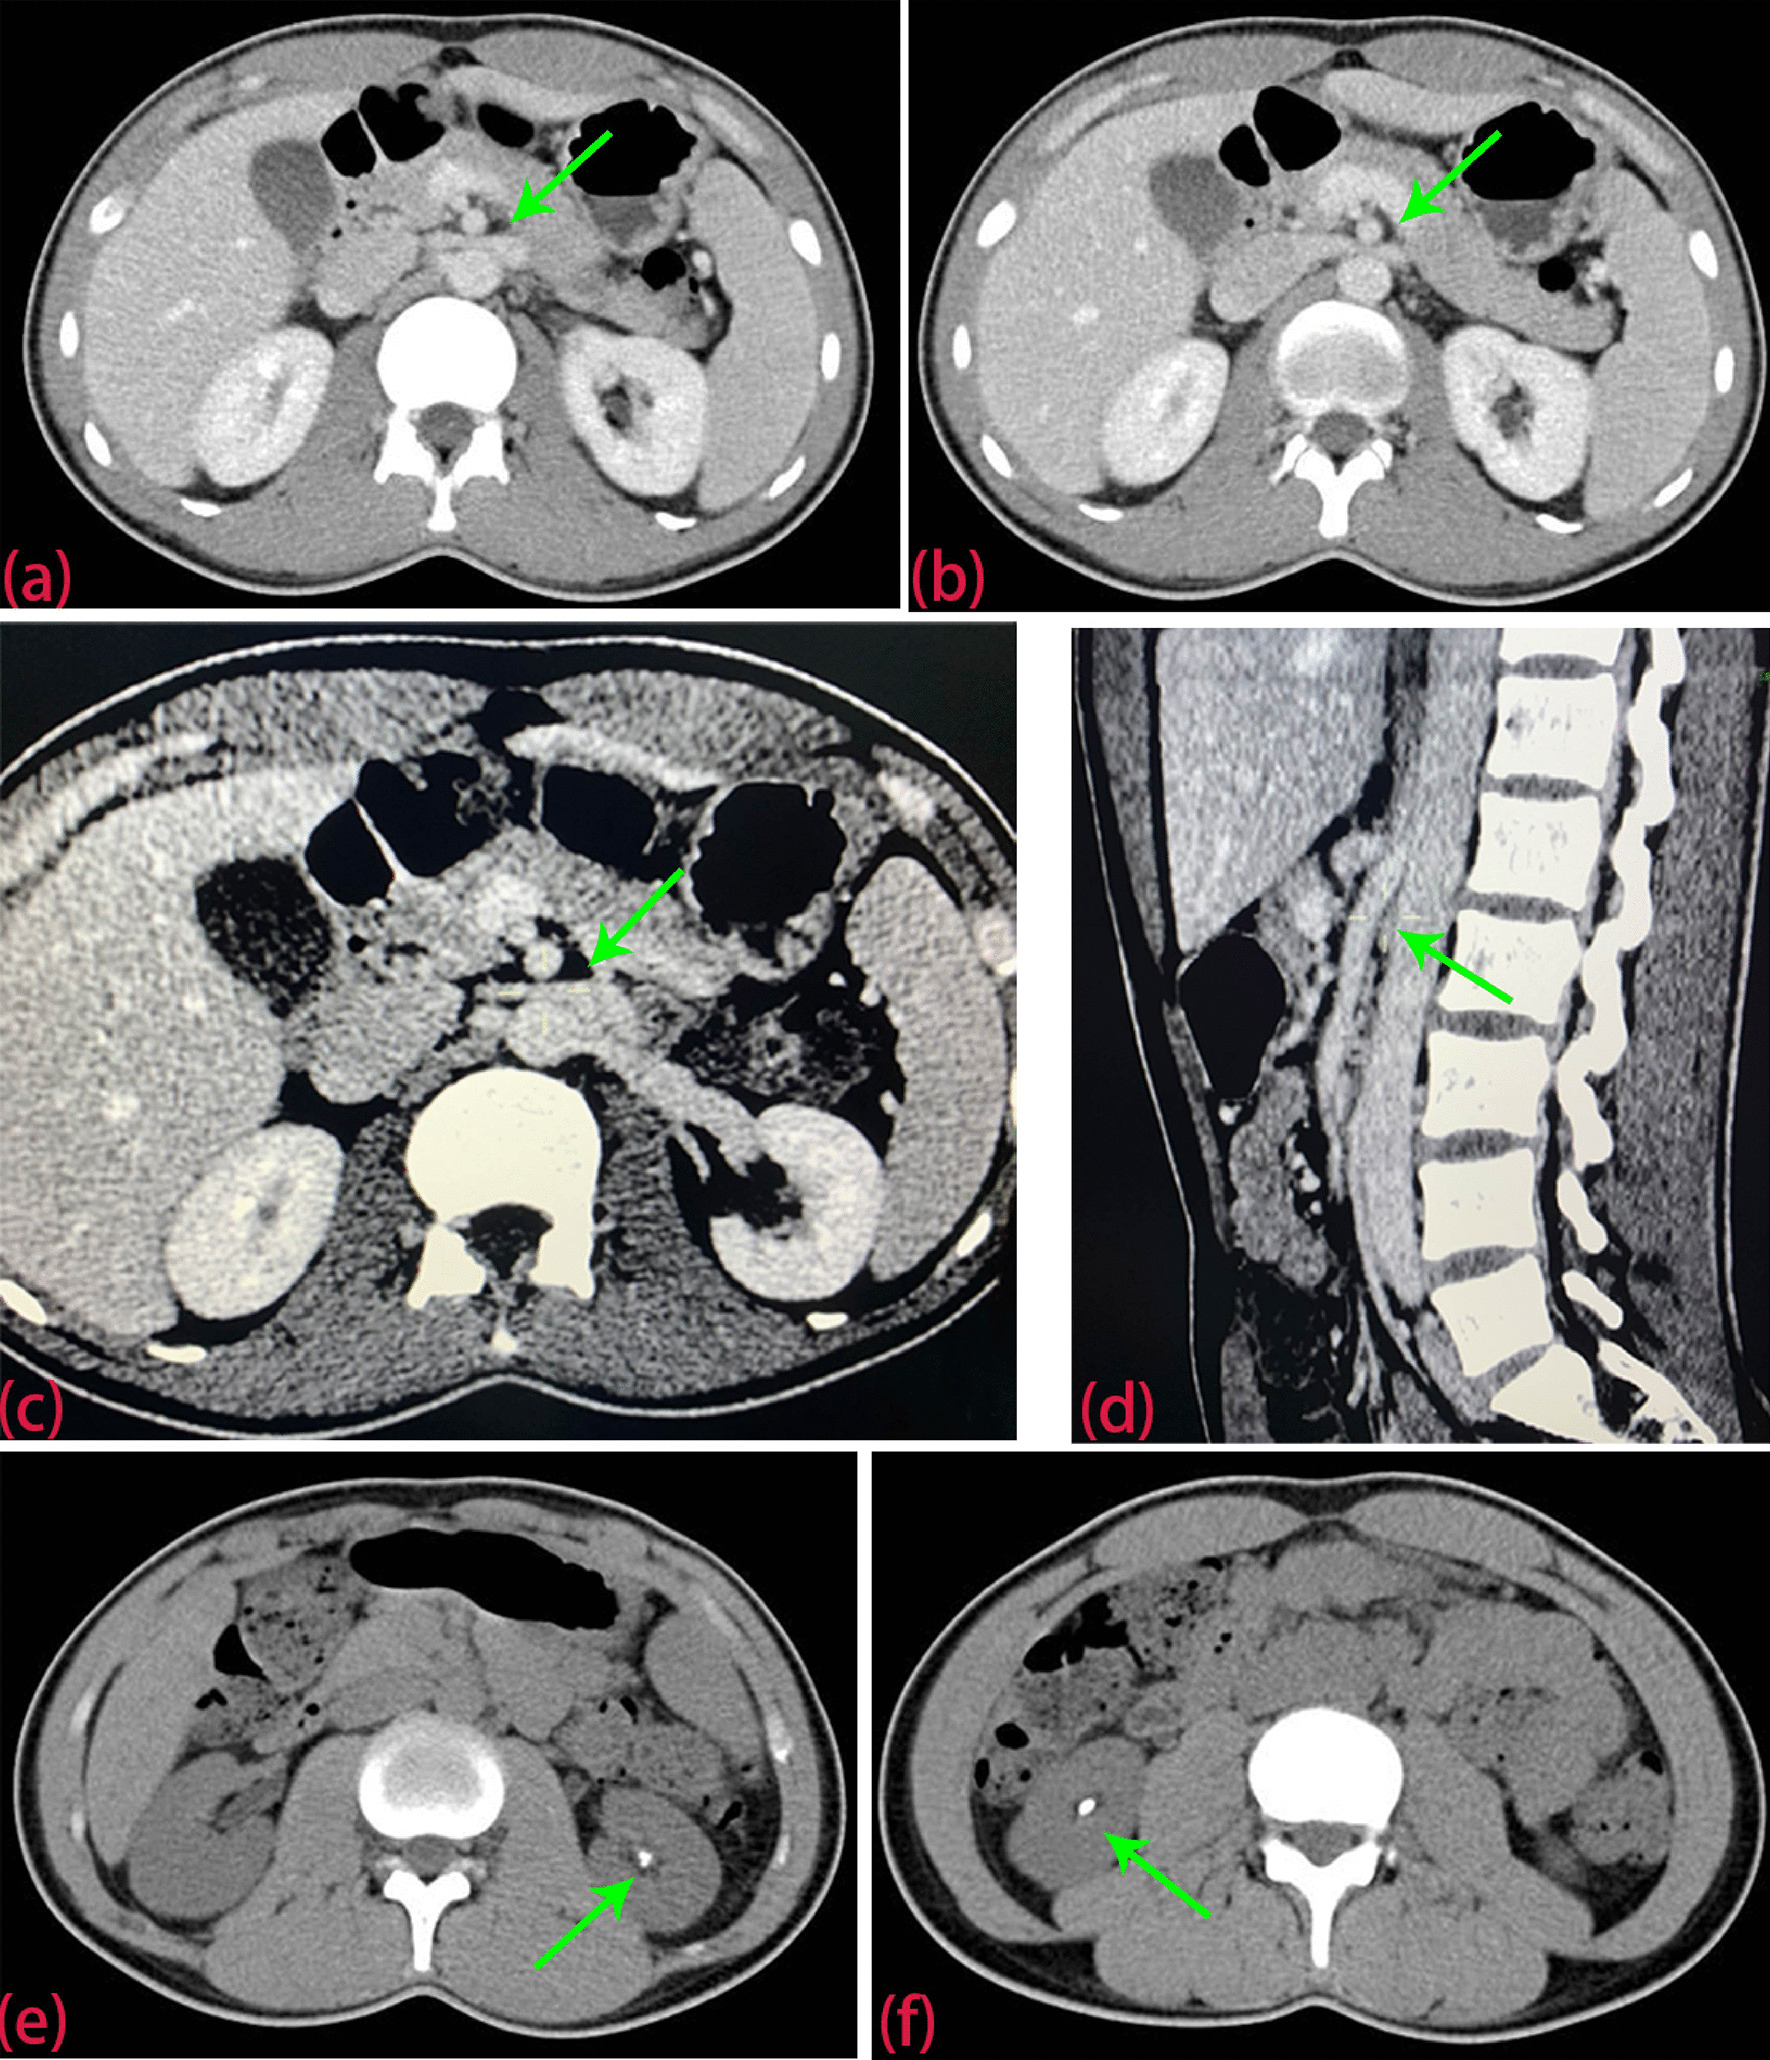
Computed tomography (CT) revealed the nutcracker phenomenon and bilateral kidney stones. a, b, c, d Abdominal CT angiography revealed that the angle between the left renal vein and the aorta had become smaller, showing the compression of left renal vein between superior mesenteric artery and abdominal aorta. e The left kidney stone. f The right kidney stone
